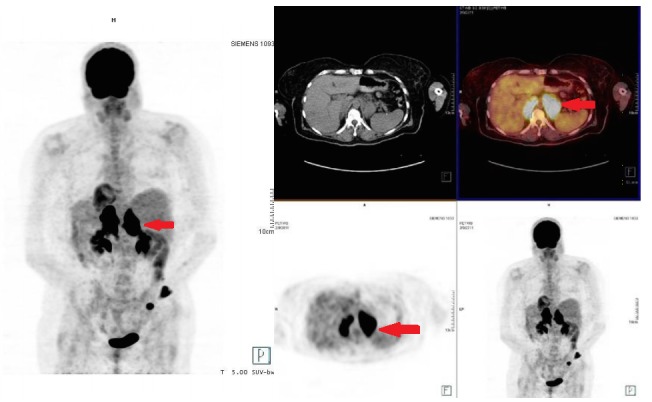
Staging FDG-PET/CT FDG-avid bilateral retroperitoneal lymphadenopathy is visualized (see arrows) indicating sites of original lymphomatous involvement.
